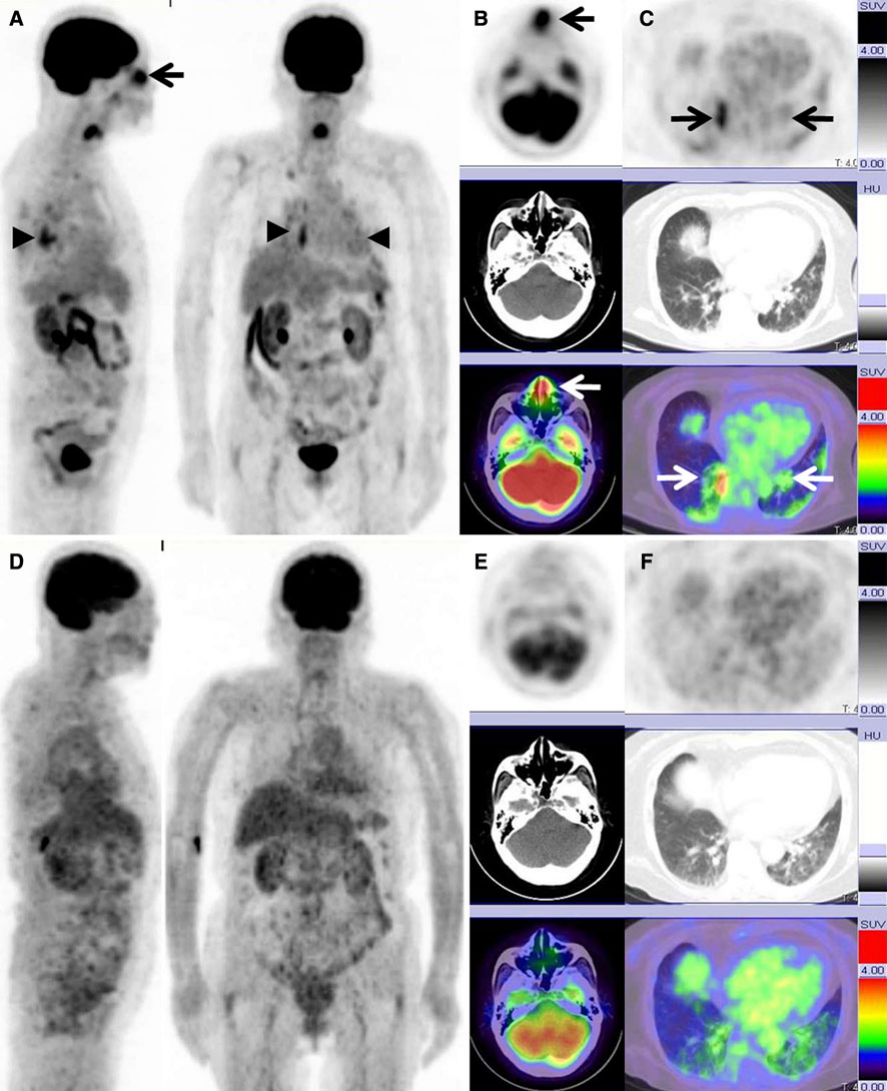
A 79-year-old woman (Case 6) underwent FDG PET/CT after developing a fever of unknown origin. Serum MPO-ANCA titer was elevated to 295 U/ml, although serum PR3-ANCA titer was within normal limits. After a biopsy performed from nasal FDG uptake area, she was diagnosed with WG. a A maximum intensity projection (MIP) image showing abnormal FDG uptake in the nasal mucosa (*arrow*), bilateral lungs (*arrow heads*), and decreased renal uptake due to renal failure. b An axial FDG PET/CT image showing increased FDG uptake in the nasal wall (arrow; max SUV, 5.10), although the findings of nonenhanced CT alone do not show the nasal lesion. c An axial FDG PET/CT image showing increased FDG uptake at the nodular shadows in both lungs (*white and black arrows*; max SUV, 4.00). After the predonisolone and immunosuppressant therapy, FDG PET/CT was performed to survey the malignancy. The patient had type 2 diabetes mellitus due to steroid therapy. d MIP images obtained after treatment show no FDG uptake in the nasal mucosa and mild FDG uptake in bilateral lungs. e, f Axial FDG PET/CT images obtained after treatment show no FDG uptake in the nasal mucosa (max SUV, 1.62) and lungs (max SUV: 2.12). The both lung lesions resisted antibiotic therapy. Finally we diagnosed these lung opacities as one of the findings on WG on the basis of the treatment response
